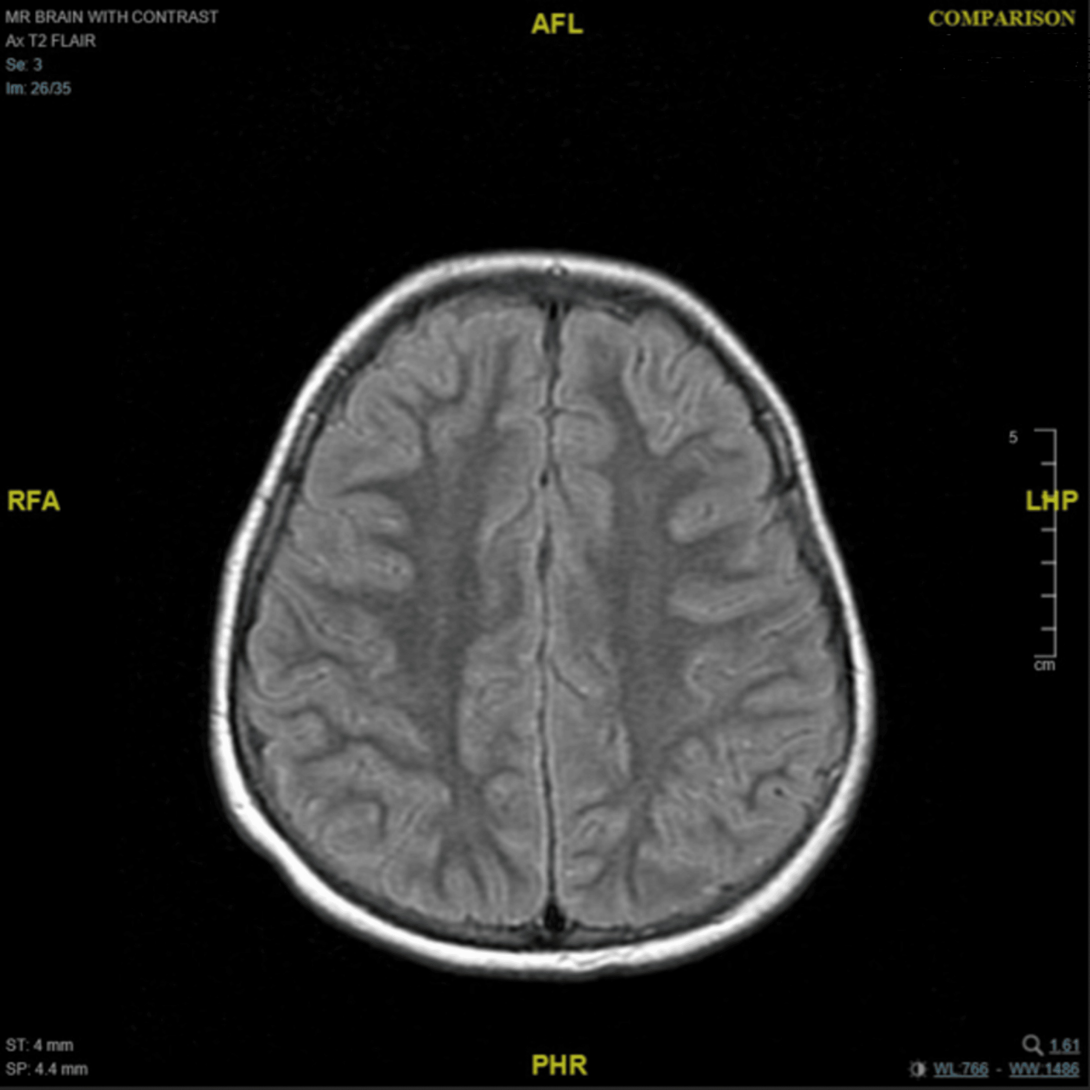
Axial T2/FLAIR brain MRI shows no definite structural or signal abnormalities FLAIR: fluid-attenuated inversion recovery; MRI: magnetic resonance imaging; T2: T2-weighted imaging; AFL: anterior-foot-left; RFA: right-foot-anterior; LHP: left-head-posterior; PHR: posterior-head-right
